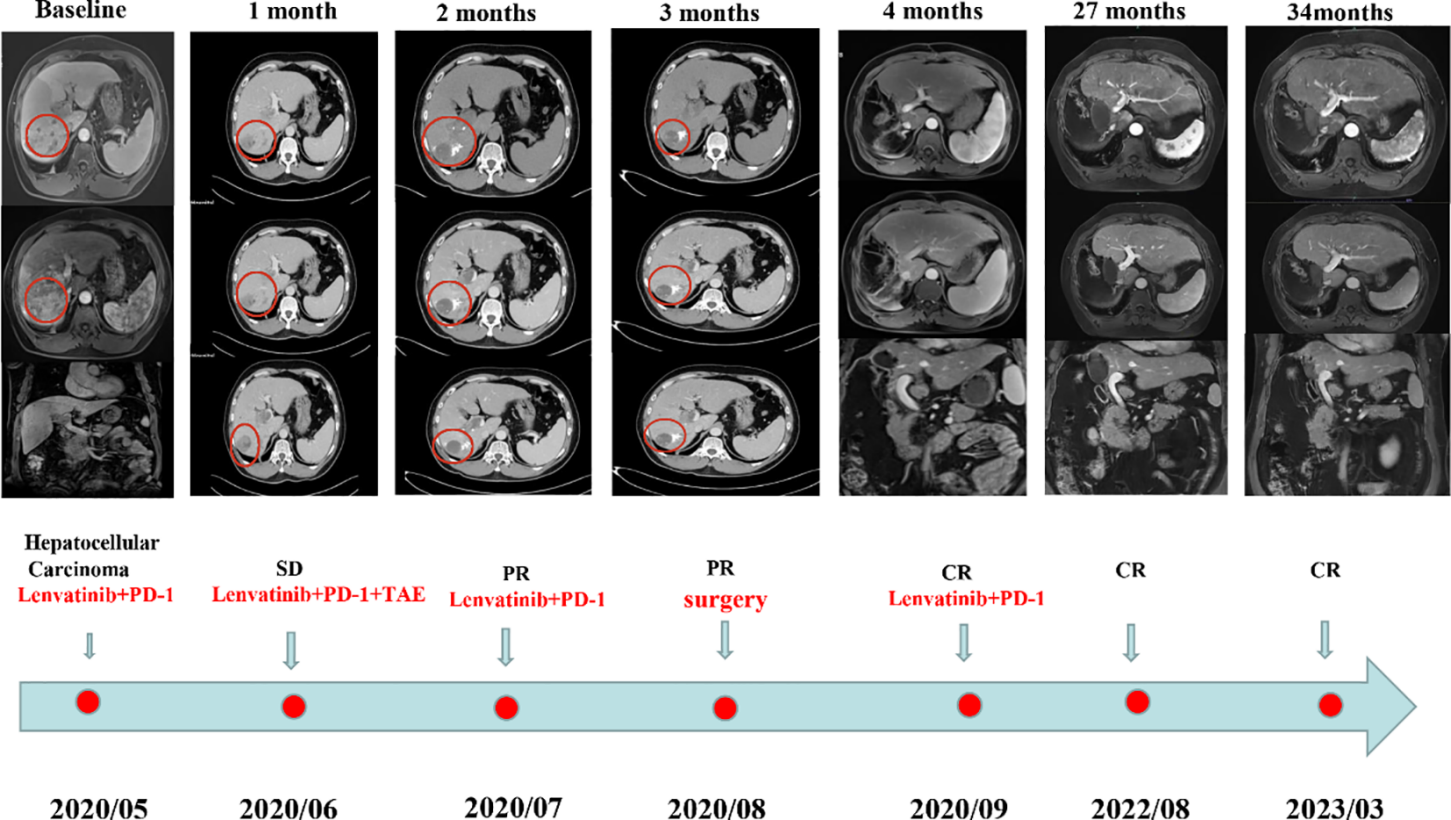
The details of the subsequent clinical course. Baseline: MRI examination revealed a lesion of approximately 1098cm in size in the right liver lobe segments S7 and S6, as well as a main portal vein thrombus.; 1 month: After three weeks of combined targeted therapy and immunotherapy, the patient’s tumor was stable according to mRECIST; 2 months: After the patient received the second round of combination targeted and immunotherapy, the response was assessed as partial response (PR); 3 months: After 4 weeks of TACE treatment, the treatment response was reassessed and again determined to be PR; 4 months: After undergoing another round of combined targeted and immunotherapy, the response was assessed as a complete response (CR); 27 months and 34 months: The patient was treated with the original regimen for six months after surgery and has been without recurrence for over 27months and 34 months.
